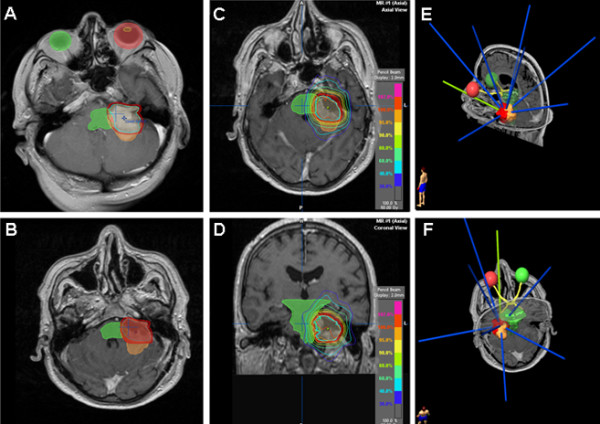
A)preoperative MRI (tumor brown, CTV blue, PTV red), B)postoperative MRI (tumor brown, CTV blue, PTV red),C)axial and D) coronal MRI showing radiation plan with isodose lines, E and F) non-coplanar and conformal arrangement of the static beams.
